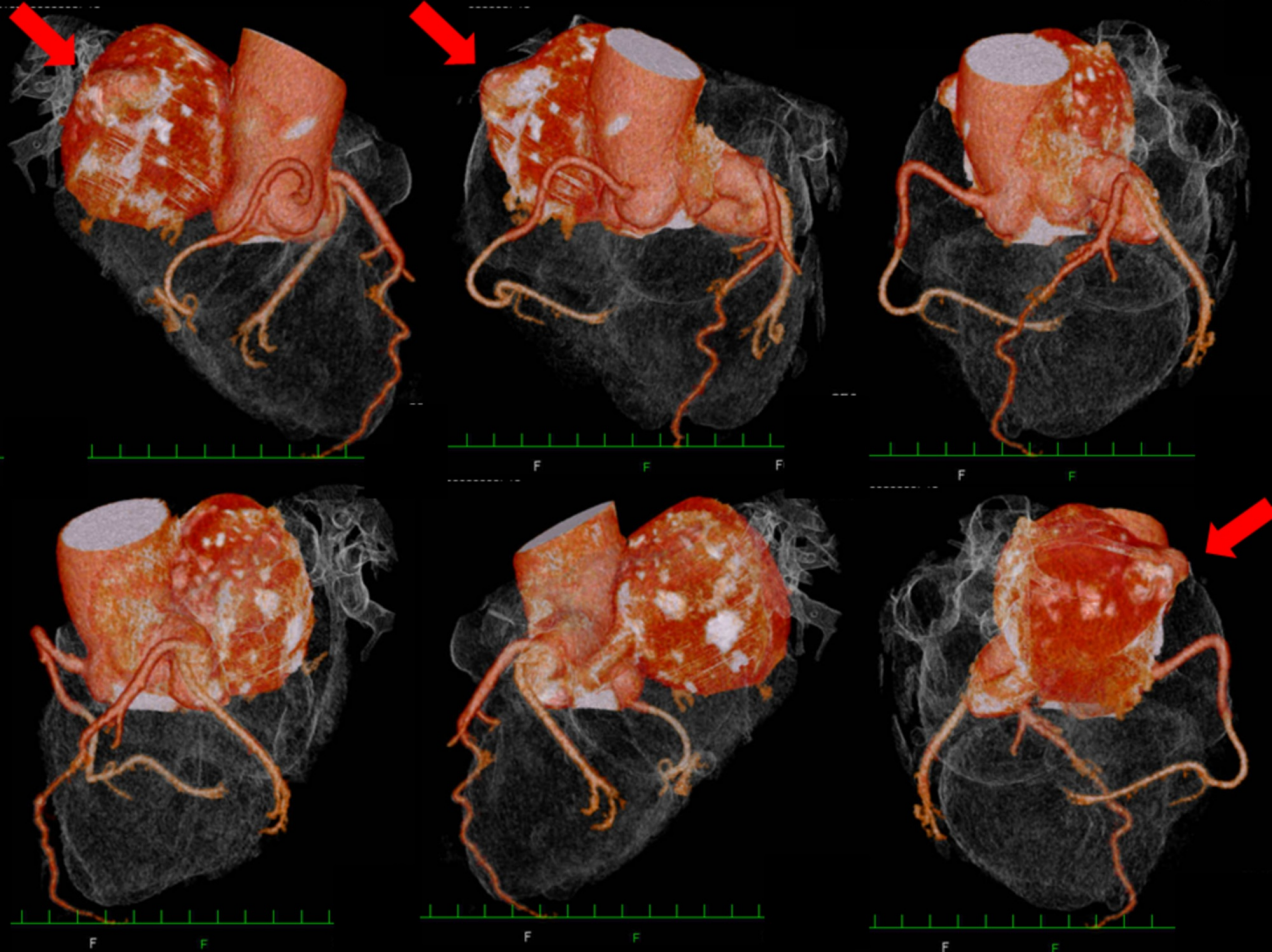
MDCT before surgery. MDCT rotational view (from top left to bottom right). Enlarged LMT branches LAD and LCX, then runs dorsally towards RA forming giant aneurysm (bottom, middle). A bulging limp (red arrow) in the aneurysm turned out to be the opening to RA. LAD, left anterior descending artery; LCX, left circumflex coronary artery; LMT, left main coronary trunk; MDCT, multidimensional computed tomography; RA, right atrium.
